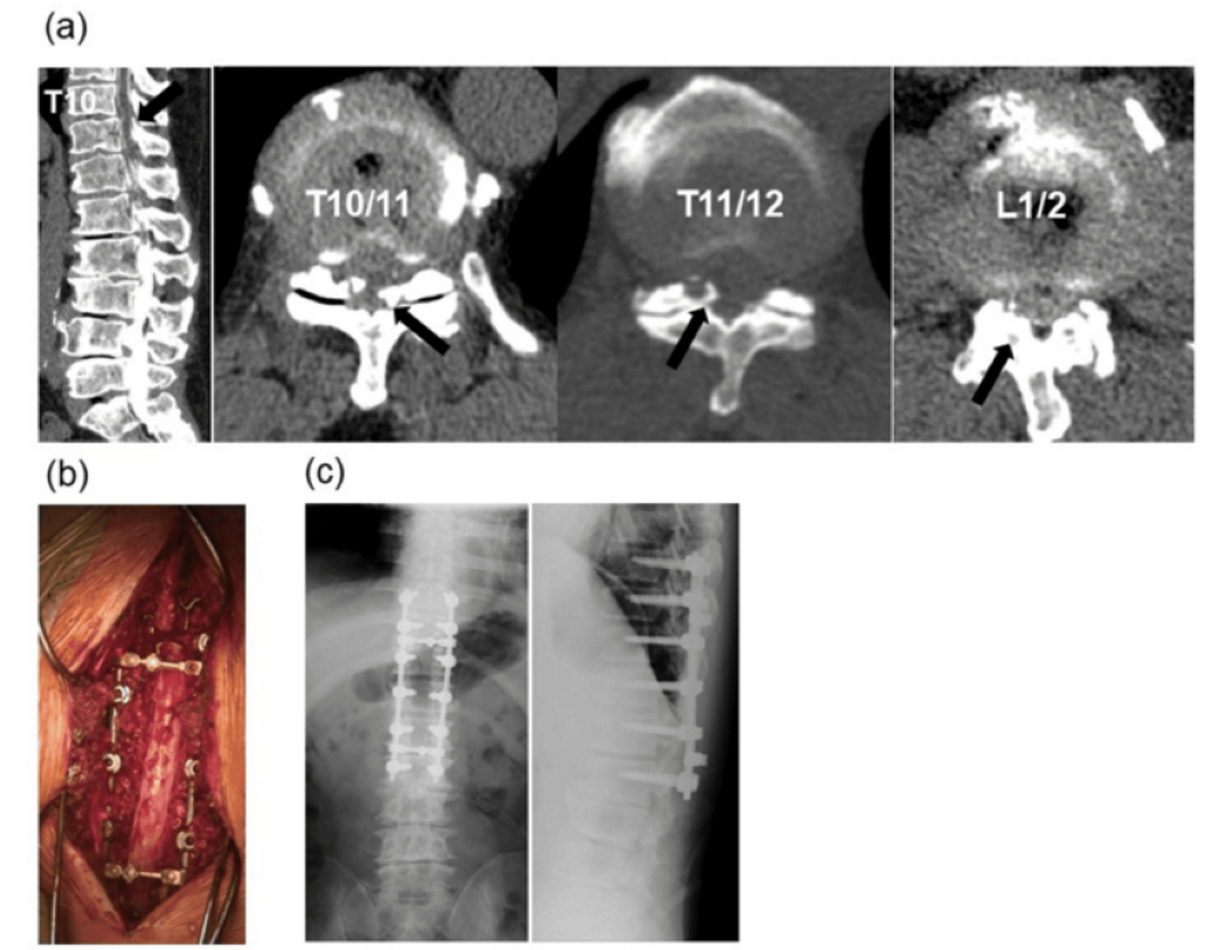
Case presentation of OLF (a) It presents preoperative OLF in the thoracolumbar level (T10/11, T11/12, and L1/2). Black arrows point to OLF. (b) It presents intraoperative image. In this case, no dural injury occurred. (c) It presents postoperative X-ray. We conducted laminectomy from T10 to L2 and a posterior fusion from T9 to L2 in this case. OLF, the ossification of the ligamentum flavum
